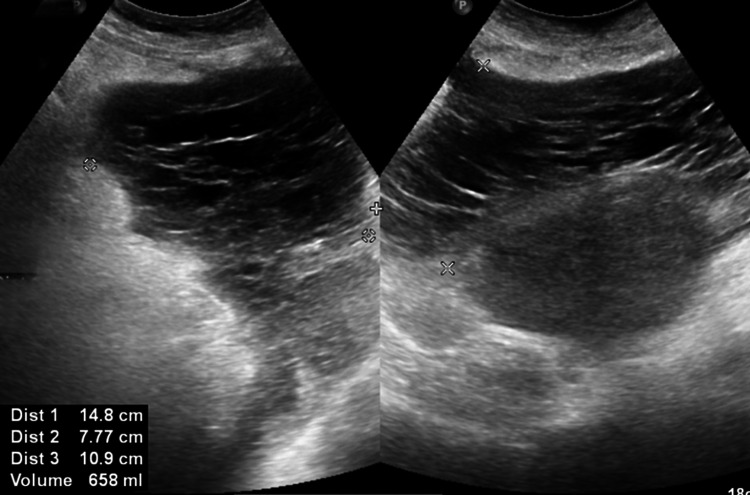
Ultrasound showing pus-filled lesion in the lower abdomen, anterior to the uterus and beneath the abdominal wall.
